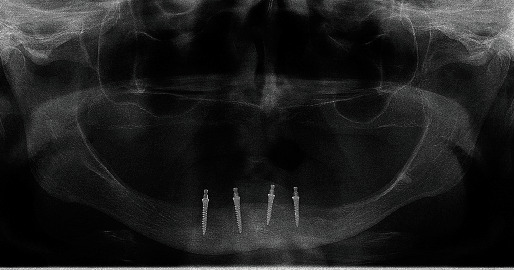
Panoramic radiogram after insertion of four MDIs.
